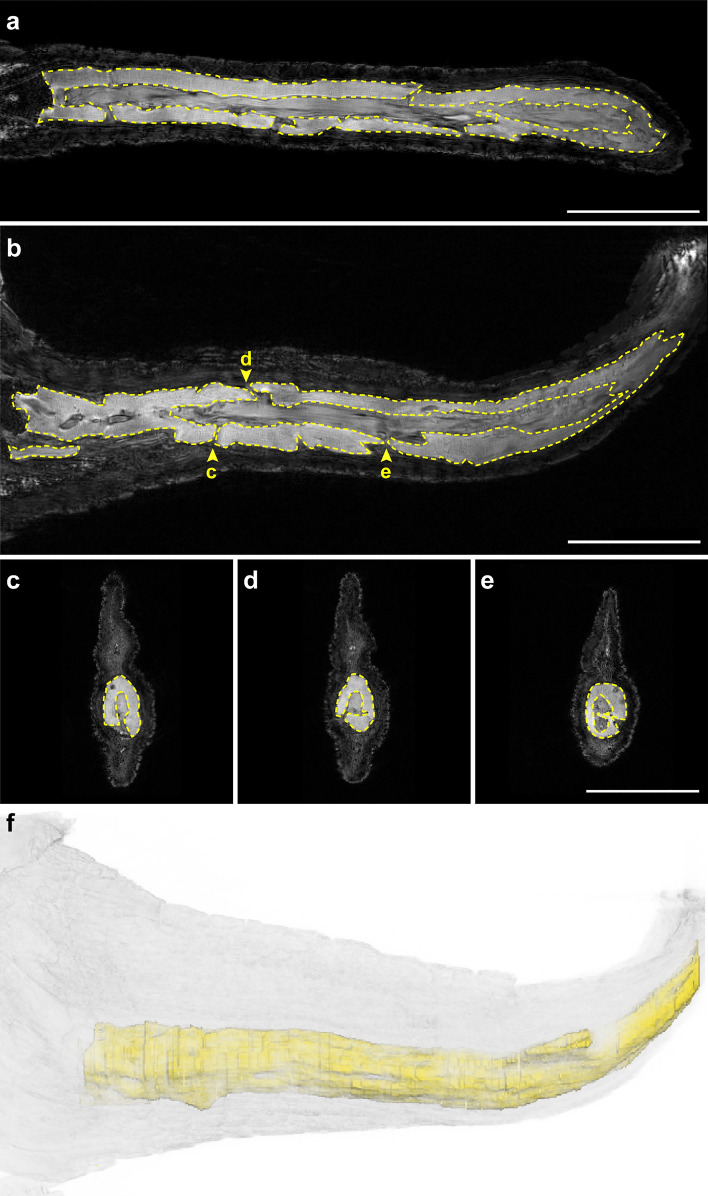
The endoskeleton of the regrown tail forms a hollow, unsegmented rod with randomly distributed foramina. Representative orthogonal magnetic resonance images (a–e) and 3D reconstruction (f) of a regrown alligator tail. The regrown endoskeleton forms a hollow tube that lacks segmentation (a,b). Foramina (b–e) are randomly distributed along the proximo-distal axis of the tail. The regrown endoskeleton is ventrally positioned in the tail (c–f). Scale bars are 2.5 cm.
